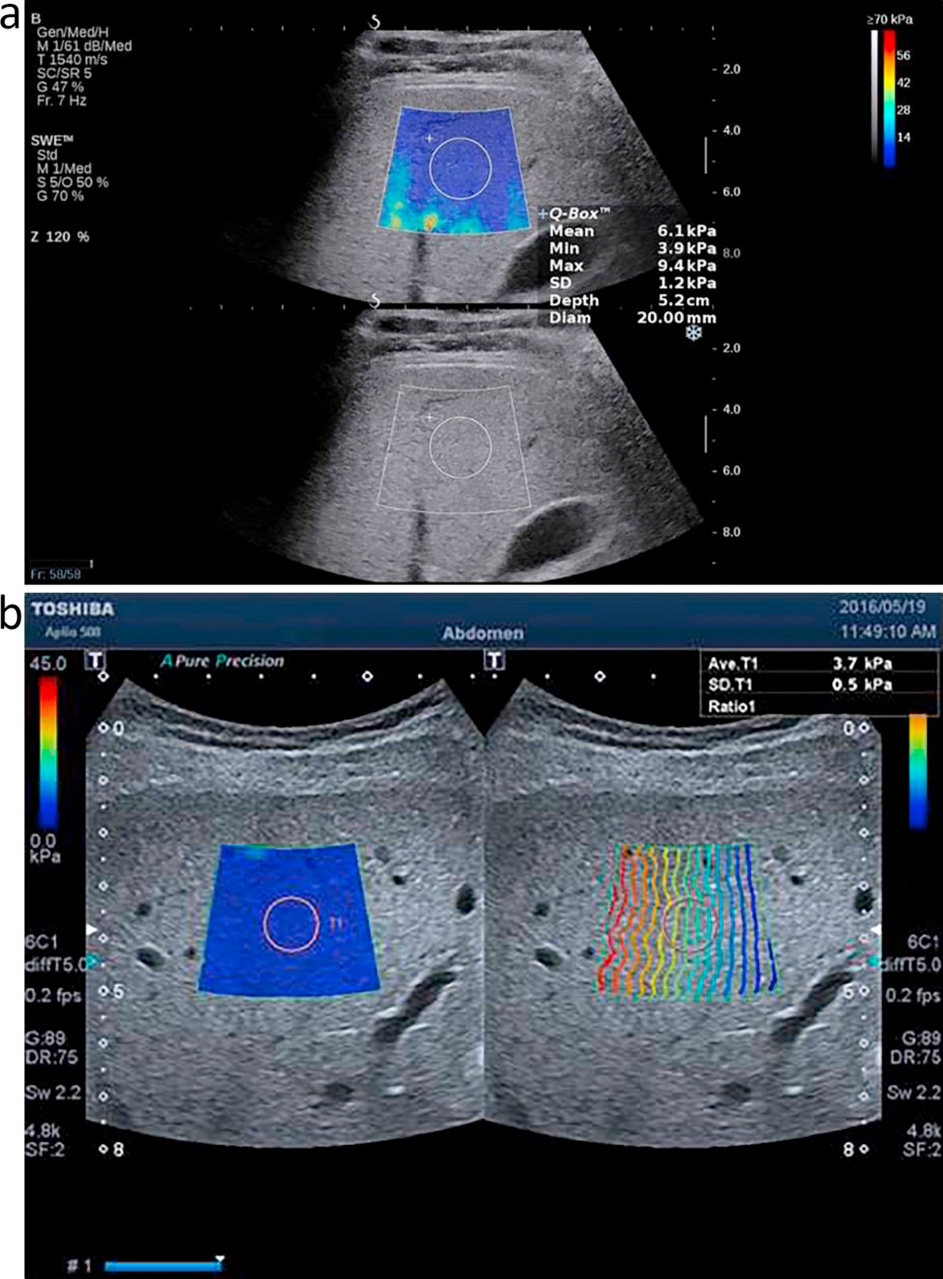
Normal liver parenchyma evaluated using 2D Shear wave elastography with different ultrasound systems. a Aixplorer (Supersonic Imagine). b Aplio 500 (Toshiba Medical Systems)
